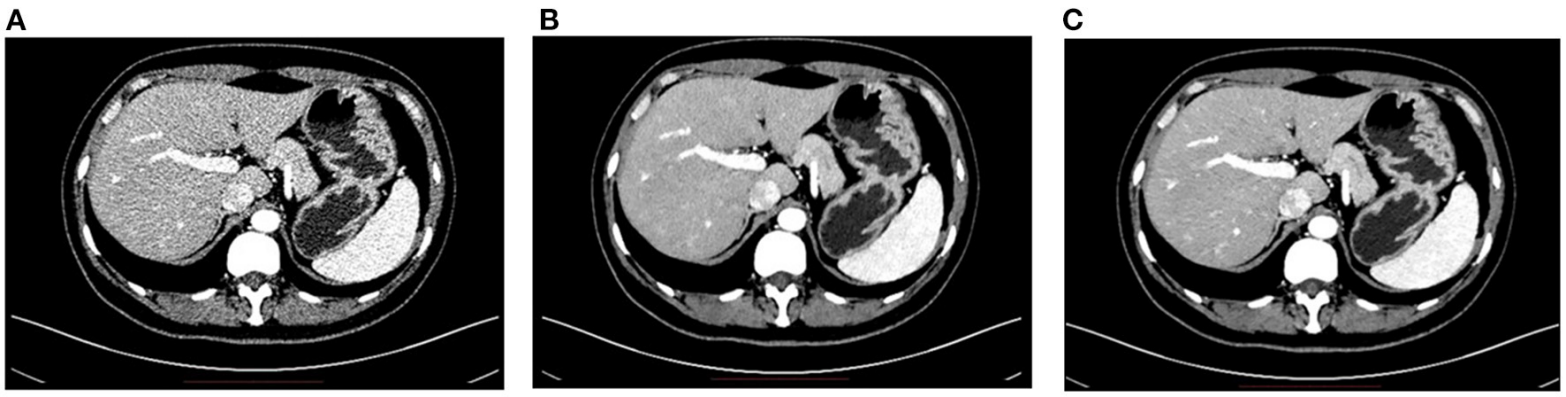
(A) The low dose abdominal CT image. (B) The resulting image after applying DELTA to the low dose image in (A). (C) The corresponding standard dose abdominal CT image.
